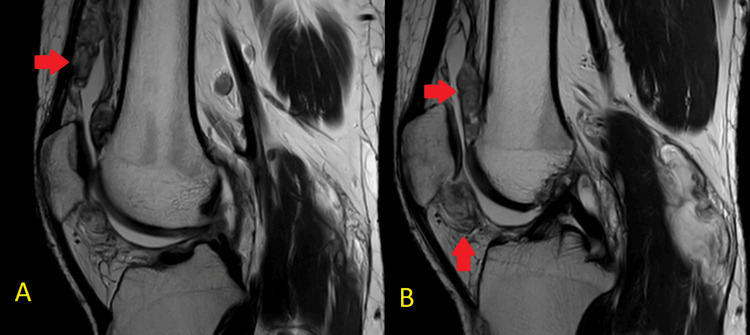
Sagittal MRI sections (A) and (B) showing multiple loose bodies T2-weighted images (A) and (B) of MRI show multiple loose bodies in the suprapatellar and infrapatellar region MRI: magnetic resonance imaging
